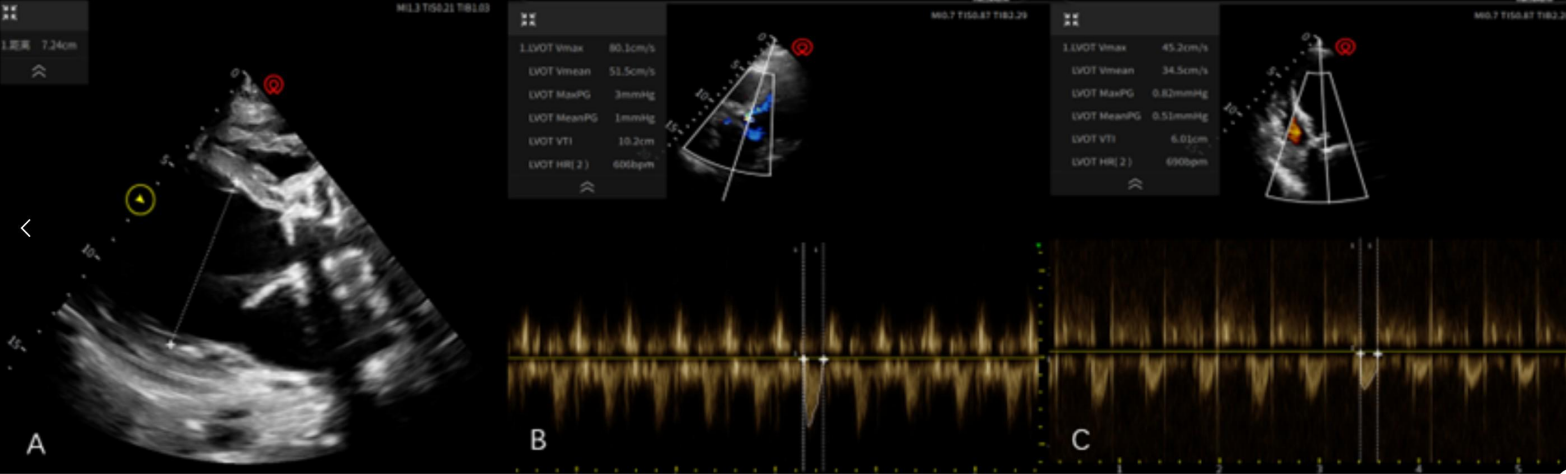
(A) Parasternal long-axis view shows diffusely weakened left ventricular contraction and severe left ventricular dilatation, with an anteroposterior left ventricular diameter of 7.2 cm; (B) aortic root velocity time integral (VTI) of 10 cm/s; (C) Aortic root velocity time integral (VTI) of 6 cm/s.
